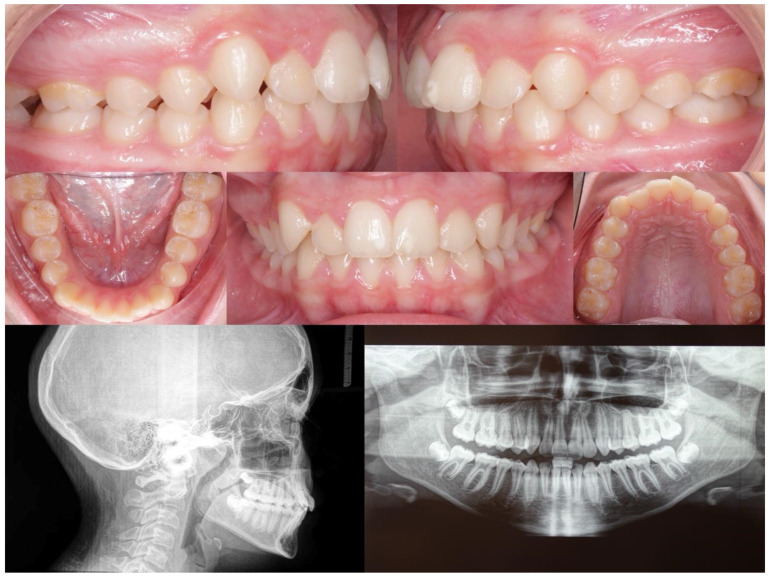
Initial right, left, front, upper, and lower orthopantomography and lateral cephalometric radiographs.
